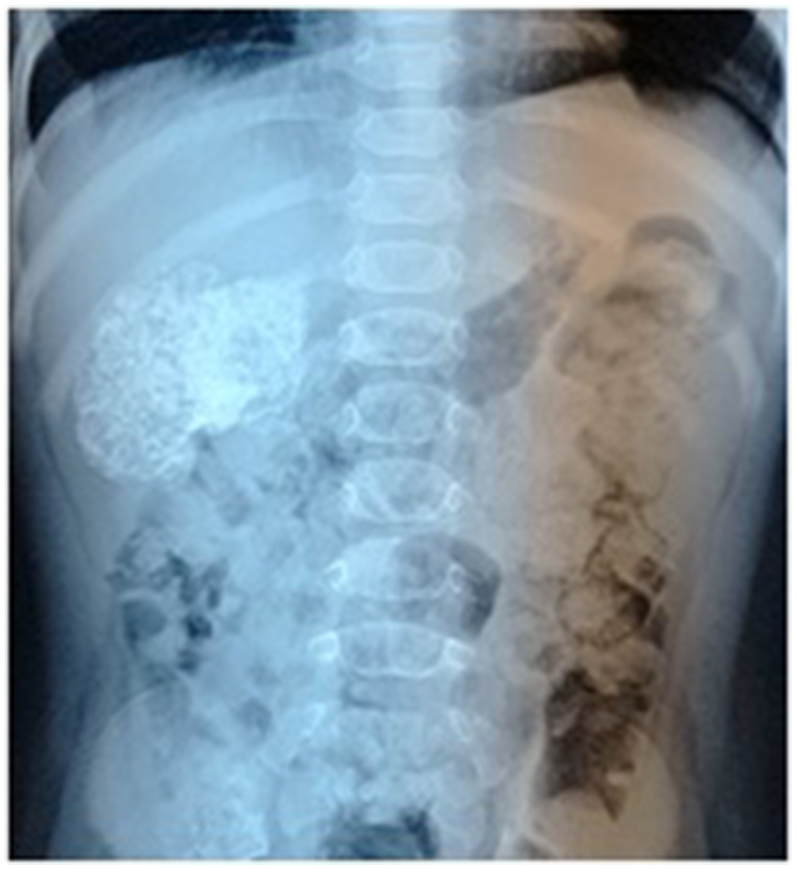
Plain radiograph shows flecks of calcifications in the expected area of the right kidney.
